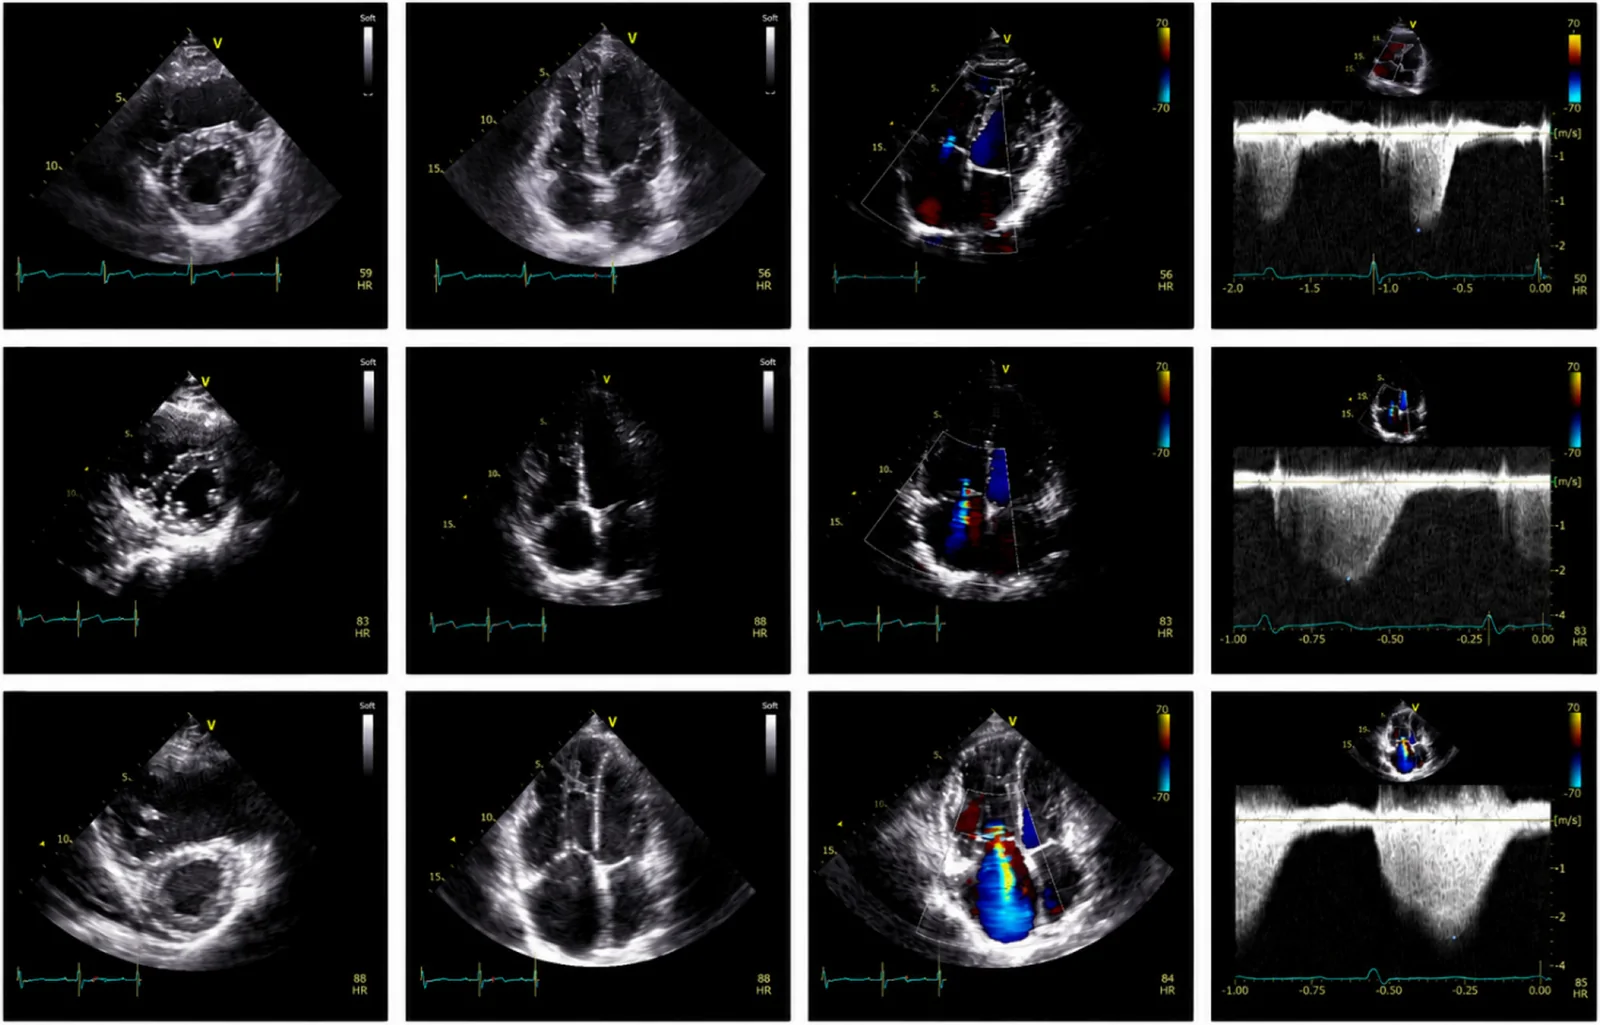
Longitudinal echocardiographic assessment of worsening pulmonary hypertension during pregnancy. Serial echocardiographic images demonstrate progressive right-sided cardiac remodeling and hemodynamic deterioration during pregnancy. This case highlights the value of serial echocardiography in monitoring right ventricular adaptation, identifying clinical deterioration, and supporting peripartum management in pregnant women with pulmonary hypertension. Panels correspond to the prepregnancy period (upper), 20 weeks of gestation (middle), and 33 weeks of gestation (bottom), respectively
